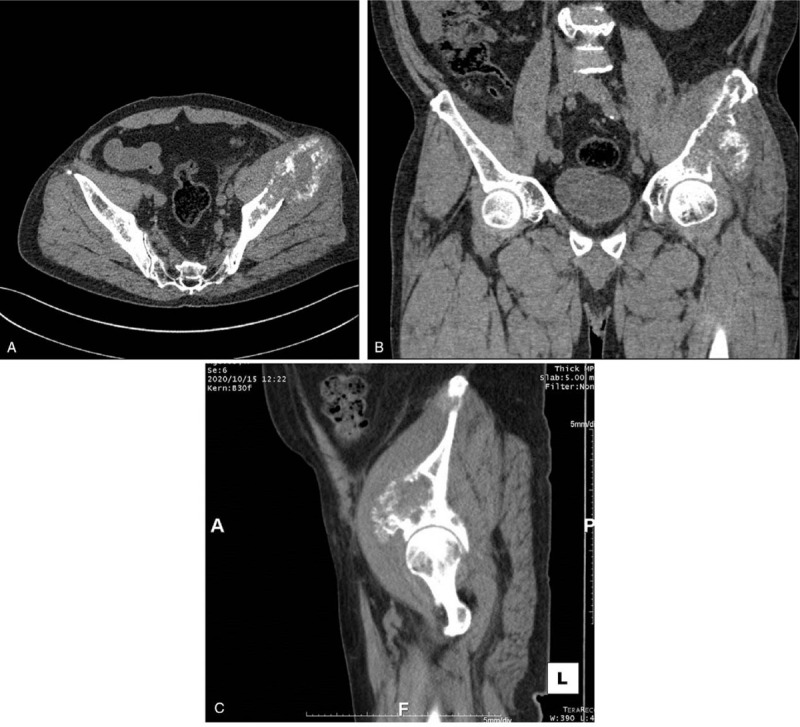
Thin-section computed tomography of the left pelvis. Horizontal view (A), coronal view (B), and sagittal view (C).
